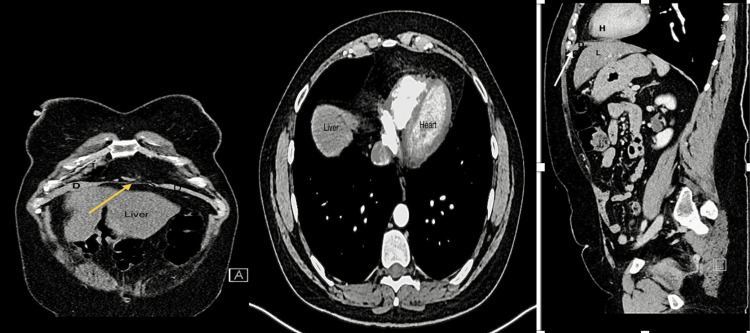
Abdominal and thoracic CT scan at six months shows no sign of diaphragmatic hernia recurrence (yellow and white arrow) D: Diaphragm, H: Heart, L: Liver
